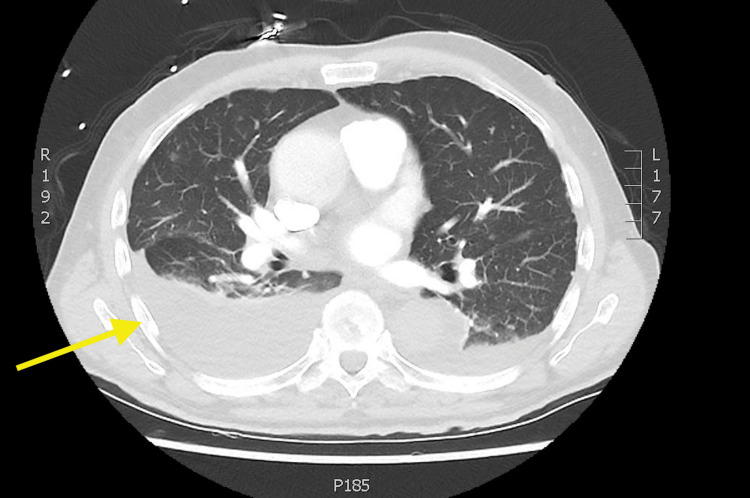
Axial CT images showing bilateral pleural effusions right worse than left CT = computed tomography
